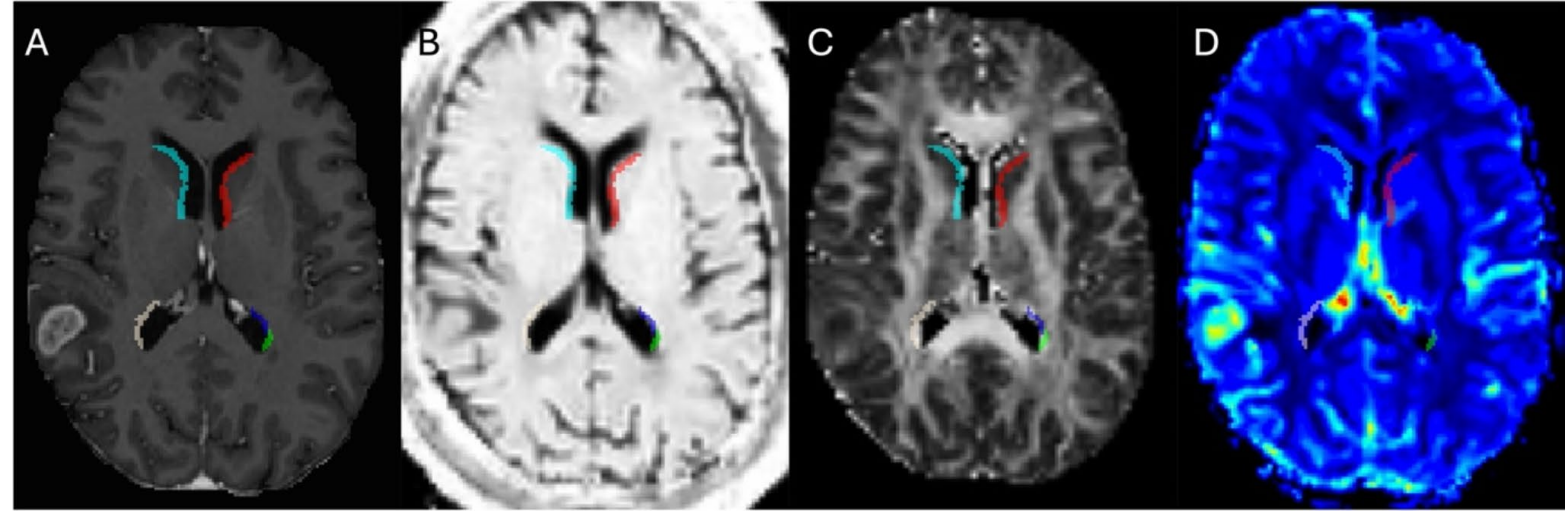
Demonstrates the segmentation of the SVZ; individual subareas of the SVZ are outlined in distinct colors using multiple MRI biomarkers: T1c (A), TVM (B), FA-FWE (C) and CBV (D)
